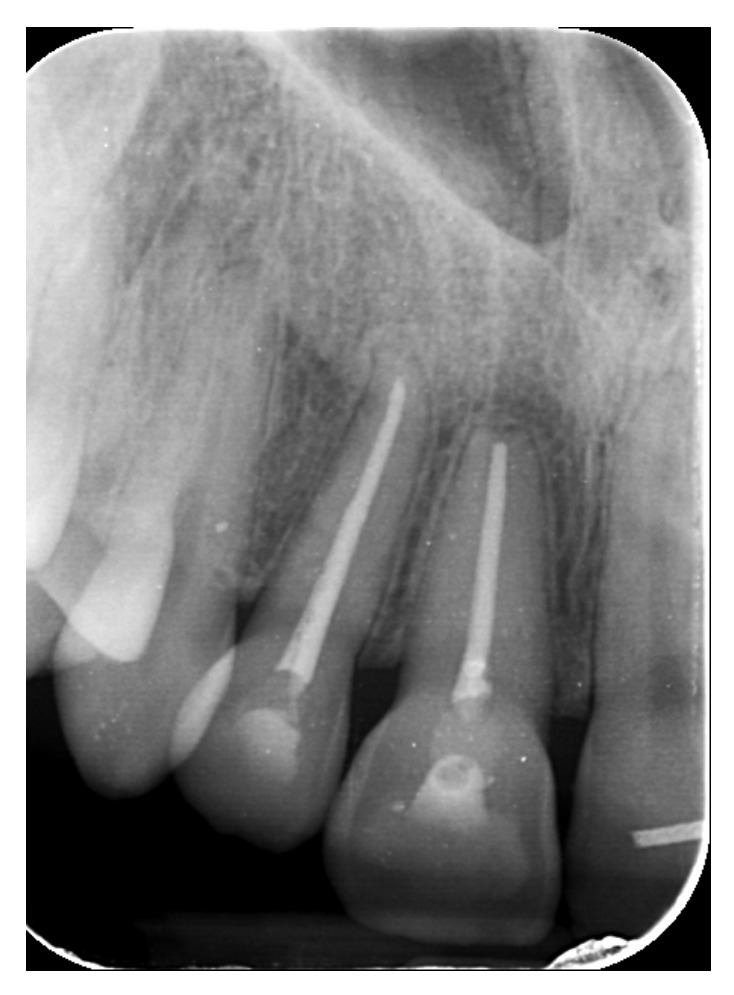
Periapical radiograph 4 years after treatment completion.
